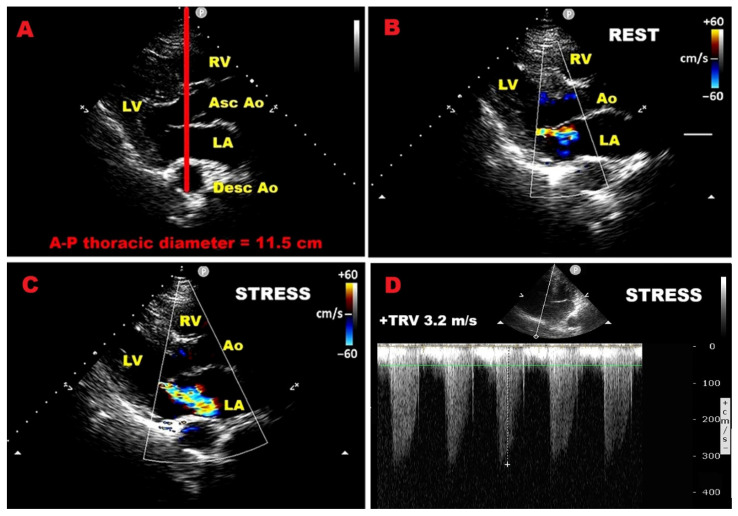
Dynamic MR assessment using ESE in symptomatic MVP with concave thorax (A-P diameter ≤ 13.5 cm) and resting mild-to-moderate MR. (A) A-P diameter measurement in the parasternal long-axis view as the distance between the true sector apex and the posterior wall of the descending aorta. (B) Parasternal long-axis view showing resting mild-to-moderate MR. (C) Parasternal long-axis view revealing peak-exercise moderate MR. (D) Continuous-wave Doppler-derived peak exercise TRV of 3.2 m/s (RV–RA gradient 42 mmHg), excluding exercise-induced severe pulmonary hypertension (sPAP < 60 mmHg). ESE, exercise stress echocardiography; MR, mitral regurgitation; MVP, mitral valve prolapse; PH, pulmonary hypertension; RA, right atrial; RV, right ventricular; sPAP, systolic pulmonary artery pressure; TRV, tricuspid regurgitation velocity.
